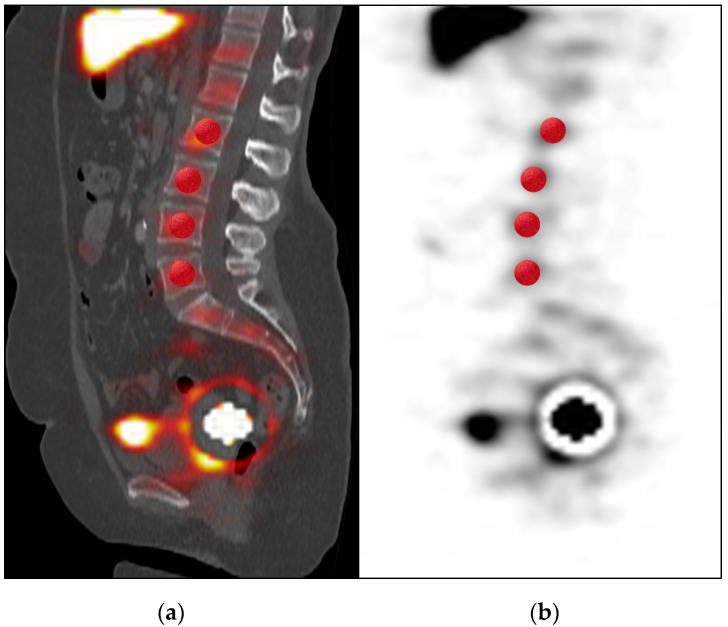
Spherical VOIs (red) placed in lumbar vertebrae (L2 to L5) shown in sagittal SPECT/CT (a) and SPECT (b) for bone marrow uptake measurement.
